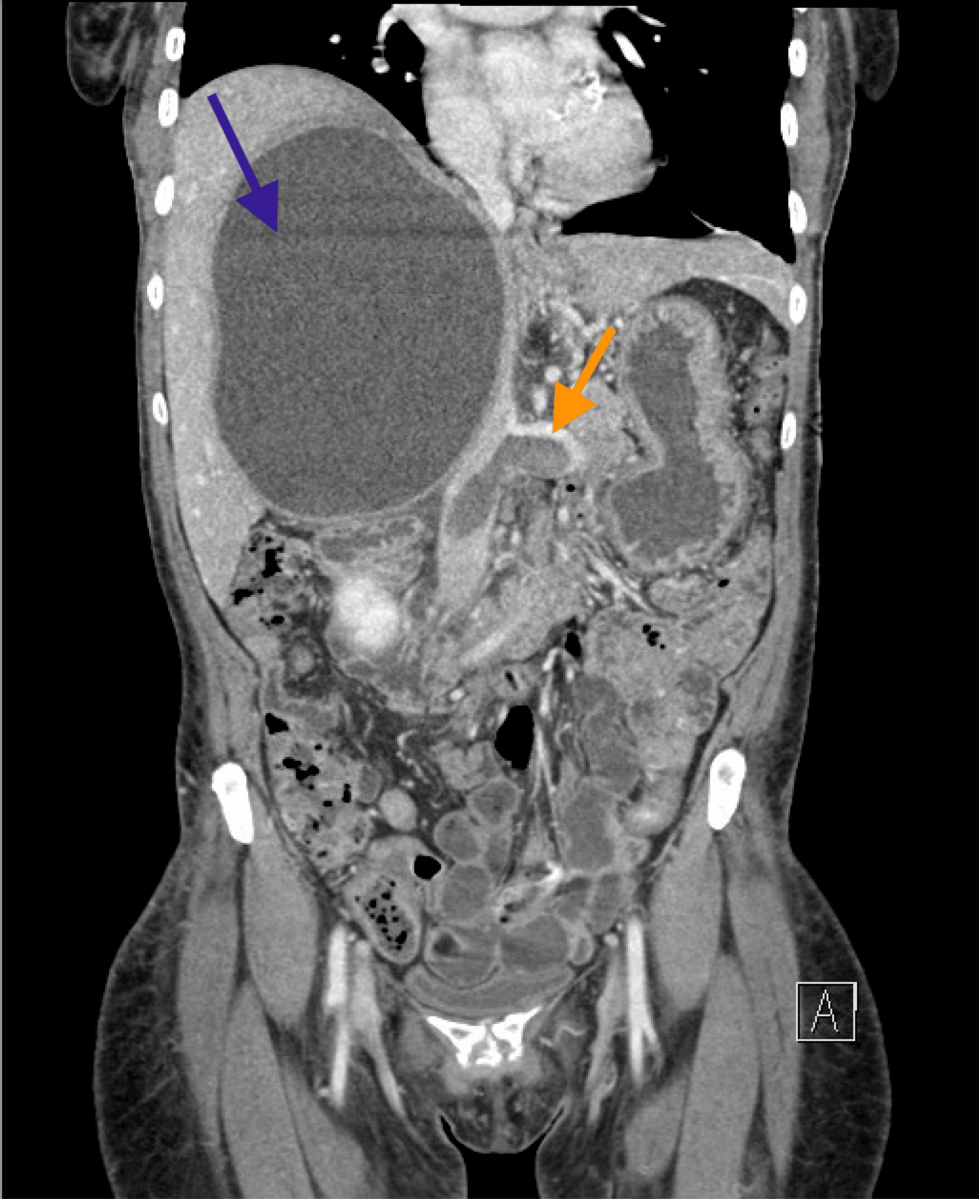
A CT of the abdomen and pelvis (venous phase) obtained on hospital day 3 The blue arrow shows a 16 cm hepatic cyst causing a mass effect on the inferior vena cava. The orange arrow indicates the extensive thrombus located below the cyst, as visualized in the inferior vena cava and left renal vein.
